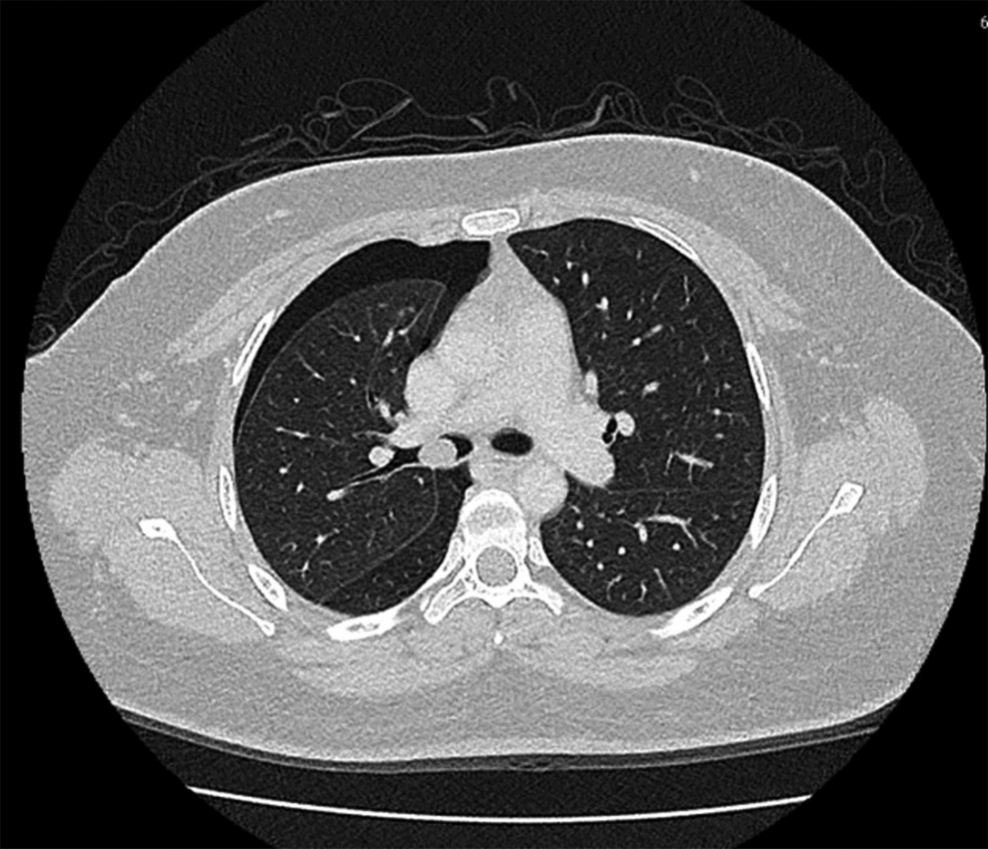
Computed tomography thorax with right endobronchial lesion and right sided pneumothorax.
